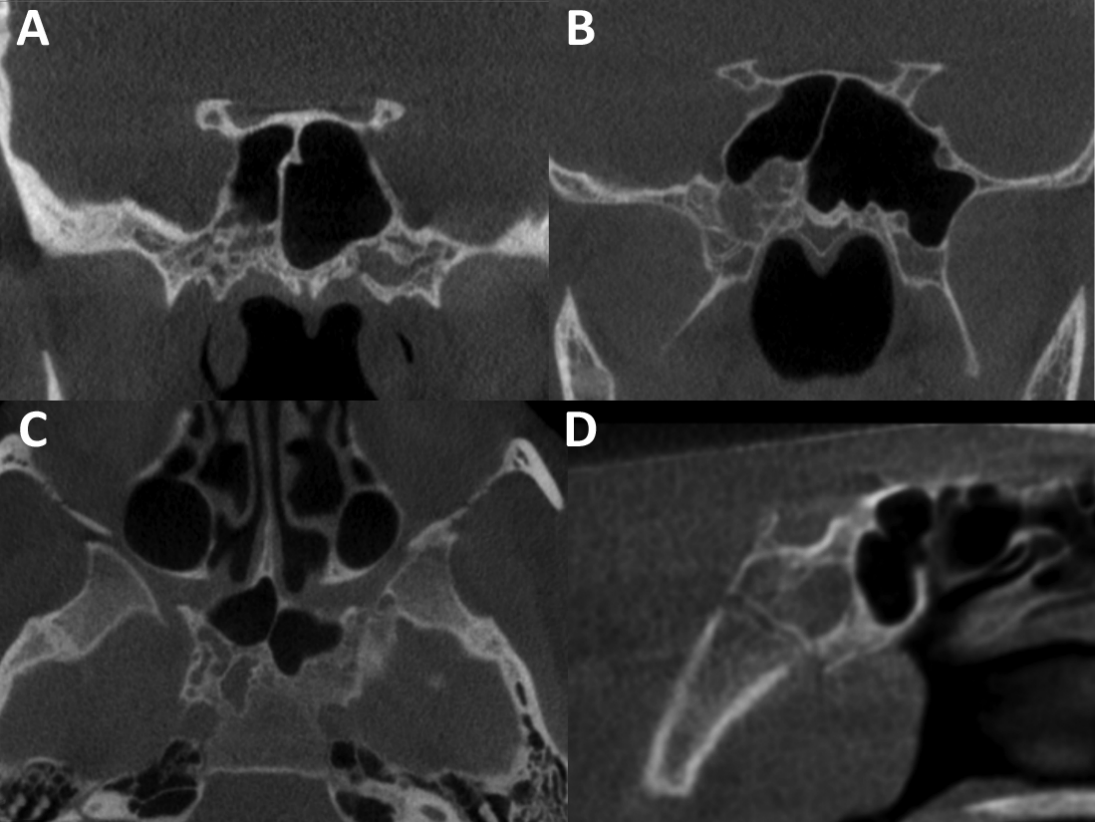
Examples of arrested pneumatization of the sphenoid (APS). Well-defined, sclerotic border, variable distributions of low-attenuation areas, and curvilinear calcifications in coronal CBCT images with (A) bilateral APS in the body and greater wings of the sphenoid, (female, 40 years), (B) right APS involving the pterygoid process (female, 13 years). (C) Axial section of APS in the right body of the sphenoid (female, 32 years). (D) Sagittal image showing unusual lack of curvilinear calcification and presence of spheno-occipital synchondrosis (male, 7 years).
